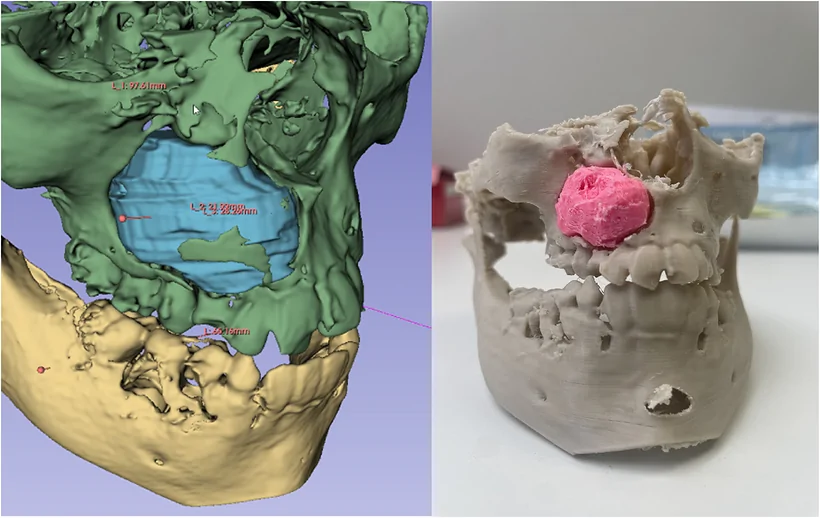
Segmentation of anatomical structures based on CBCT data and corresponding 3D‐printed model showing the mandible and maxillary cyst, with the cyst printed in soft pink PETG.
